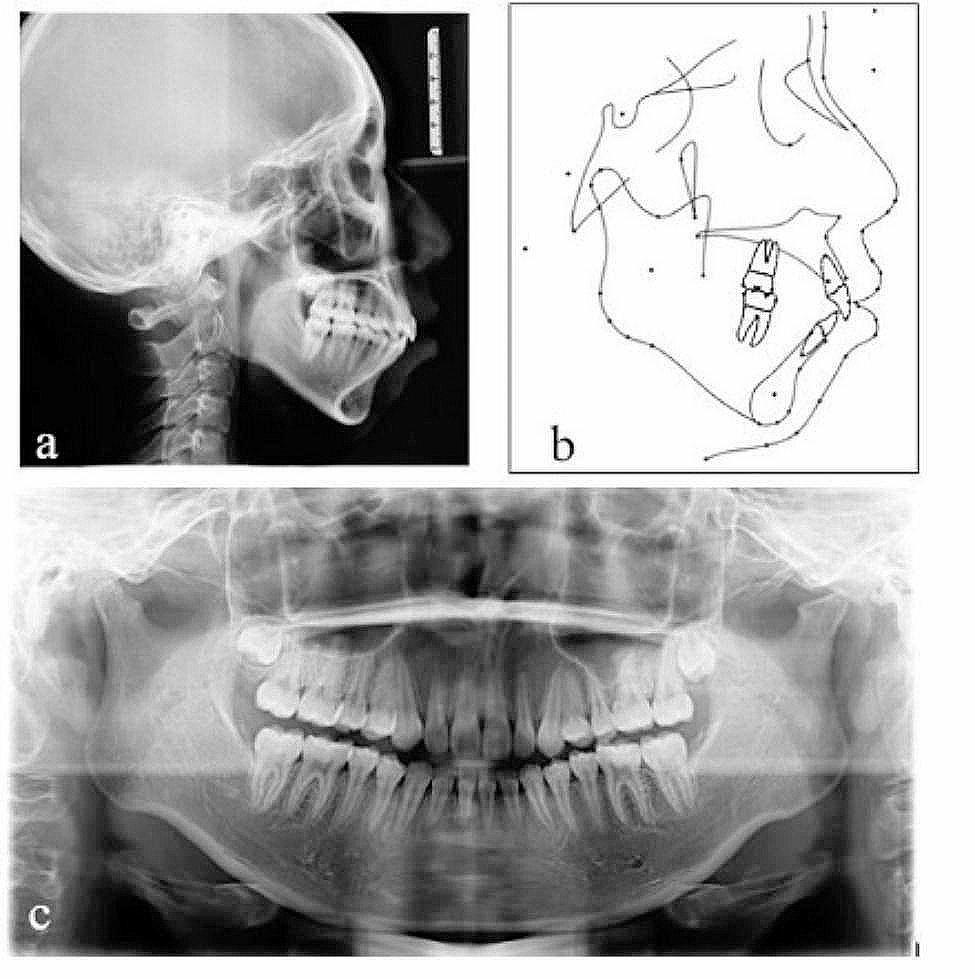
Posttreatment radiographs: a lateral cephalogram, b Pretreatment lateral cephalogram tracing, c panoramic radiograph
